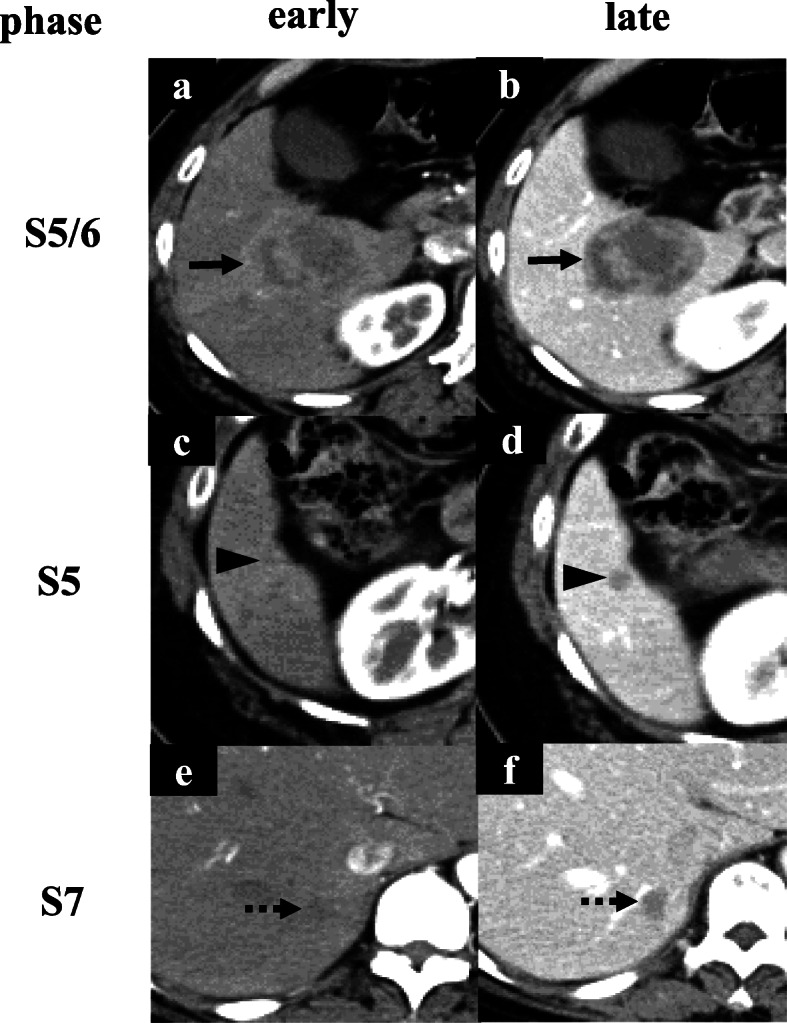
Enhanced CT (computed tomography) findings. a Enhanced CT showed a tumor with ring enhancement in S5/6 (black arrow). c, e S5 and S7 tumors were not observed in the early phase (black arrowhead, dotted black arrow). b, d, f All three tumors were visualized as low-density regions in the late phase
